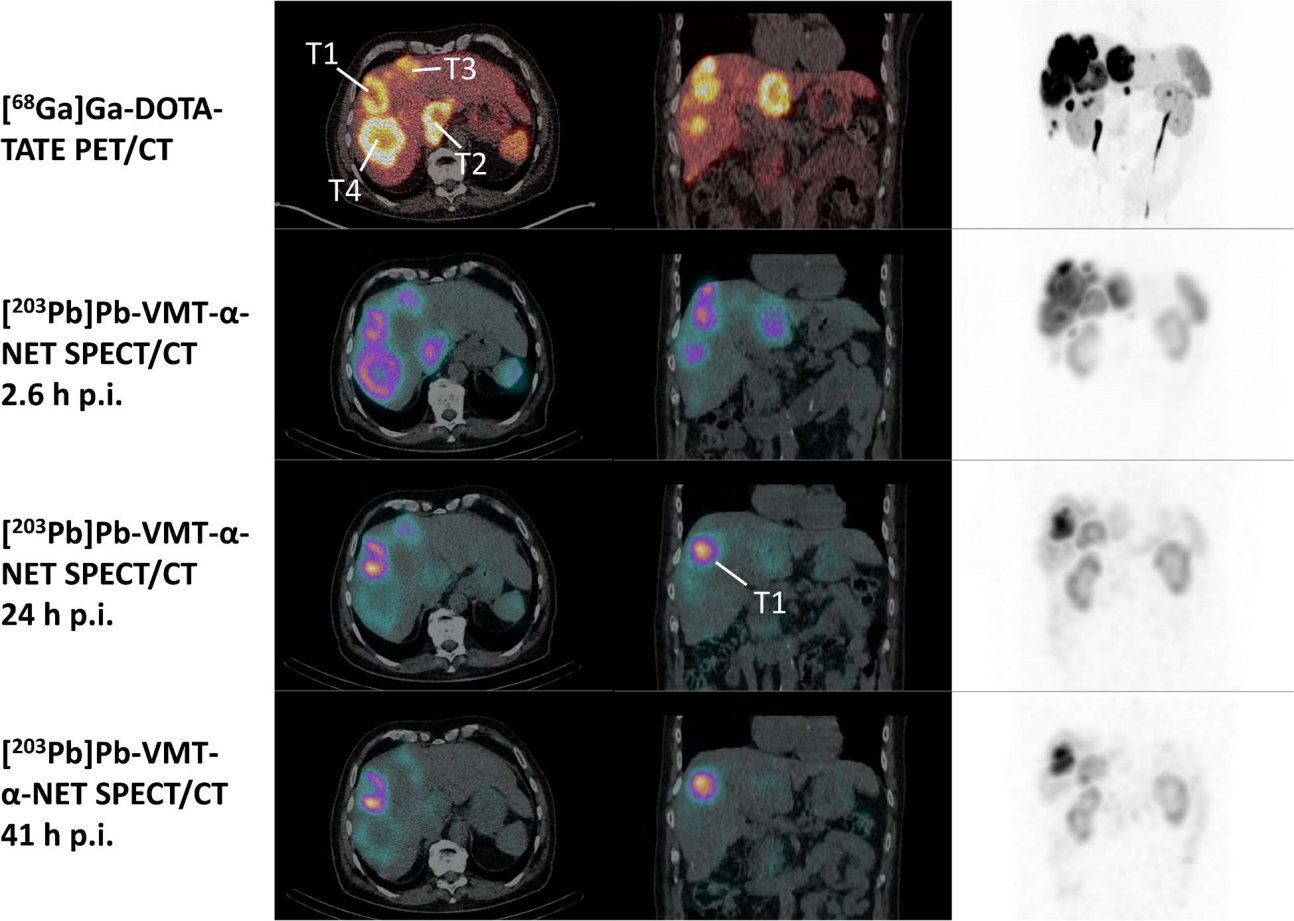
First row: Transversal and coronal slices of [^68^ Ga]Ga-DOTATATE PET/CT fusion images and maximum intensity projections (MIP) of Patient No. 2 with GEP NET G3 and multiple liver metastases before administration of [^203^Pb]Pb-VMT-α-NET. Four tumor lesions (T1-T4) were identified for dosimetry. Second to fourth row: Transversal and coronal slices of SPECT/CT images and MIP of the same patient at 2.6, 24 and 41 h of after administration of 348 MBq [^203^Pb]Pb-VMT-α-NET. Note the initial intense uptake in all tumor lesions at 2.6 h p.i. and the changing tracer accumulation in all 4 lesions over time
